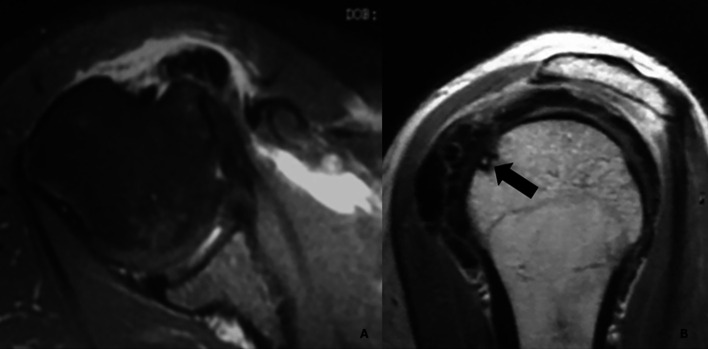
Magnetic resonance imaging of a Type III-a calcification in the subscapularis tendon.* A. Axial view showing a calcific deposit in direct contact with the cortex of the lesser tuberosity, associated with marked subcoracoid bursitis. B. Oblique sagittal view demonstrating focal cortical erosion at the site of contact (black arrow), without evidence of intraosseous extension.
